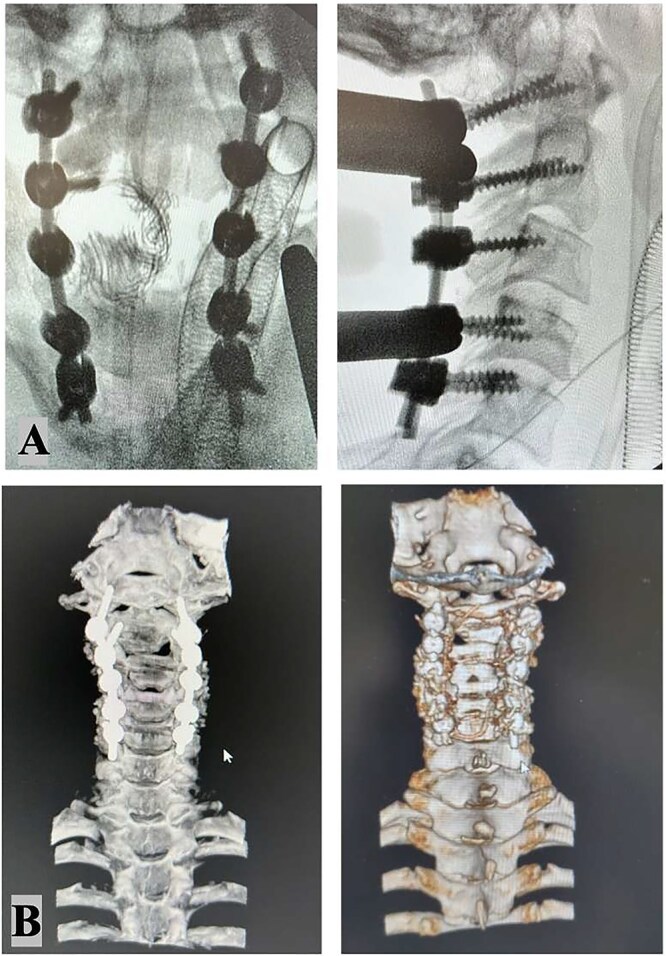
Intraoperative X-ray: Stable fixation and proper alignment of the cervical spine after C1–2–3–4–5 bilateral screw placement (A). Postoperative CT scan imaging confirmed successful realignment and structural integrity (B).
